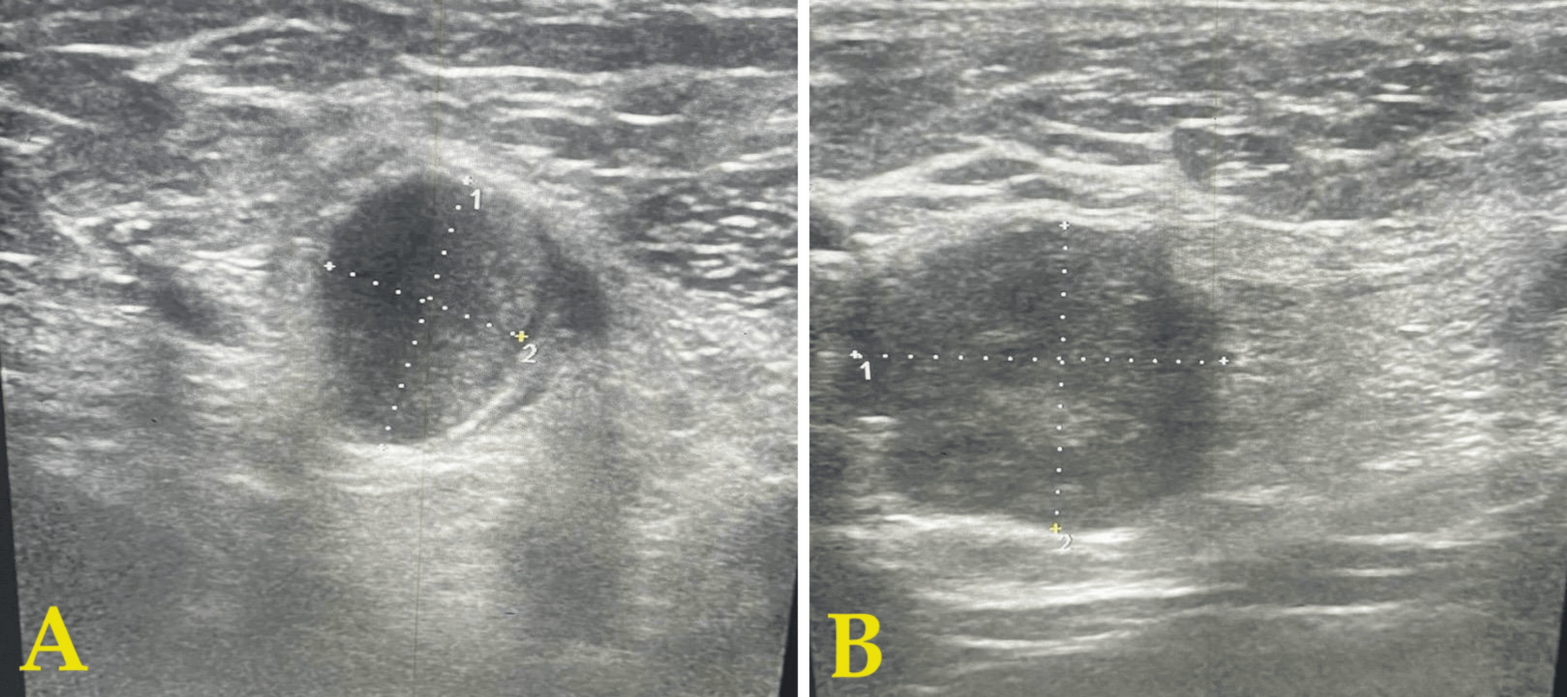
USG of the inguinoscrotal region suggestive of a right-sided inguinal hernia and right-sided edematous spermatic cord in (A) normal and (B) zoomed-in views USG: ultrasound imaging
